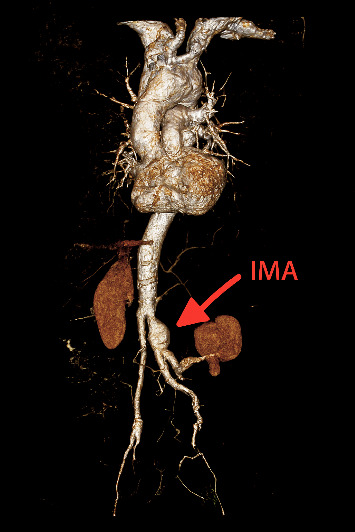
CTA 3D reconstruction showing ruptured IMA aneurysm and associated abdominal aortic dissection (red arrow).
